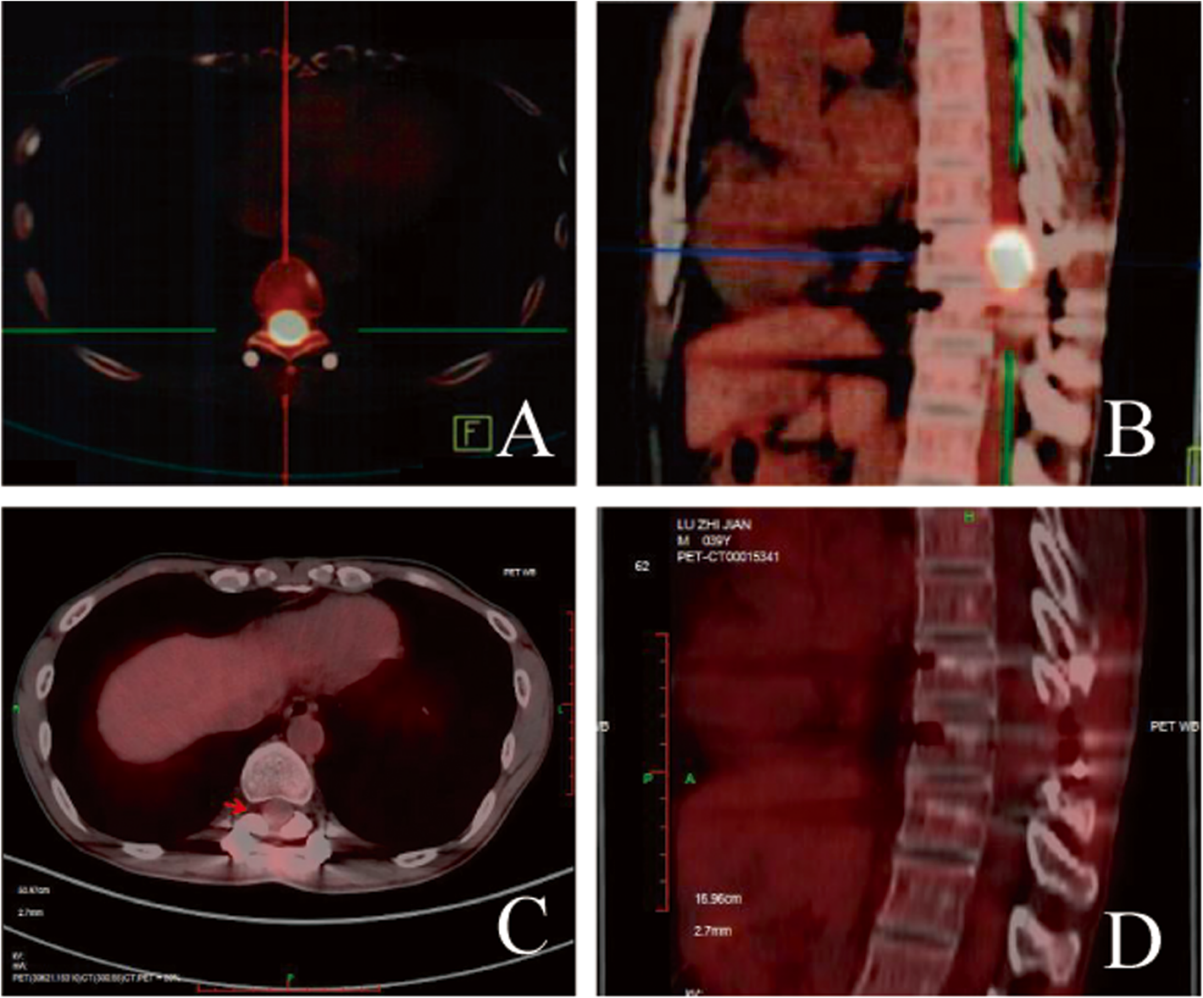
Positron emission tomography-computed tomography (PET-CT) scans showing the second recurrence in the 10th thoracic spinal cord on April 17th, 2017 (a and b), and complete response on August 24th, 2018 (c and d)
